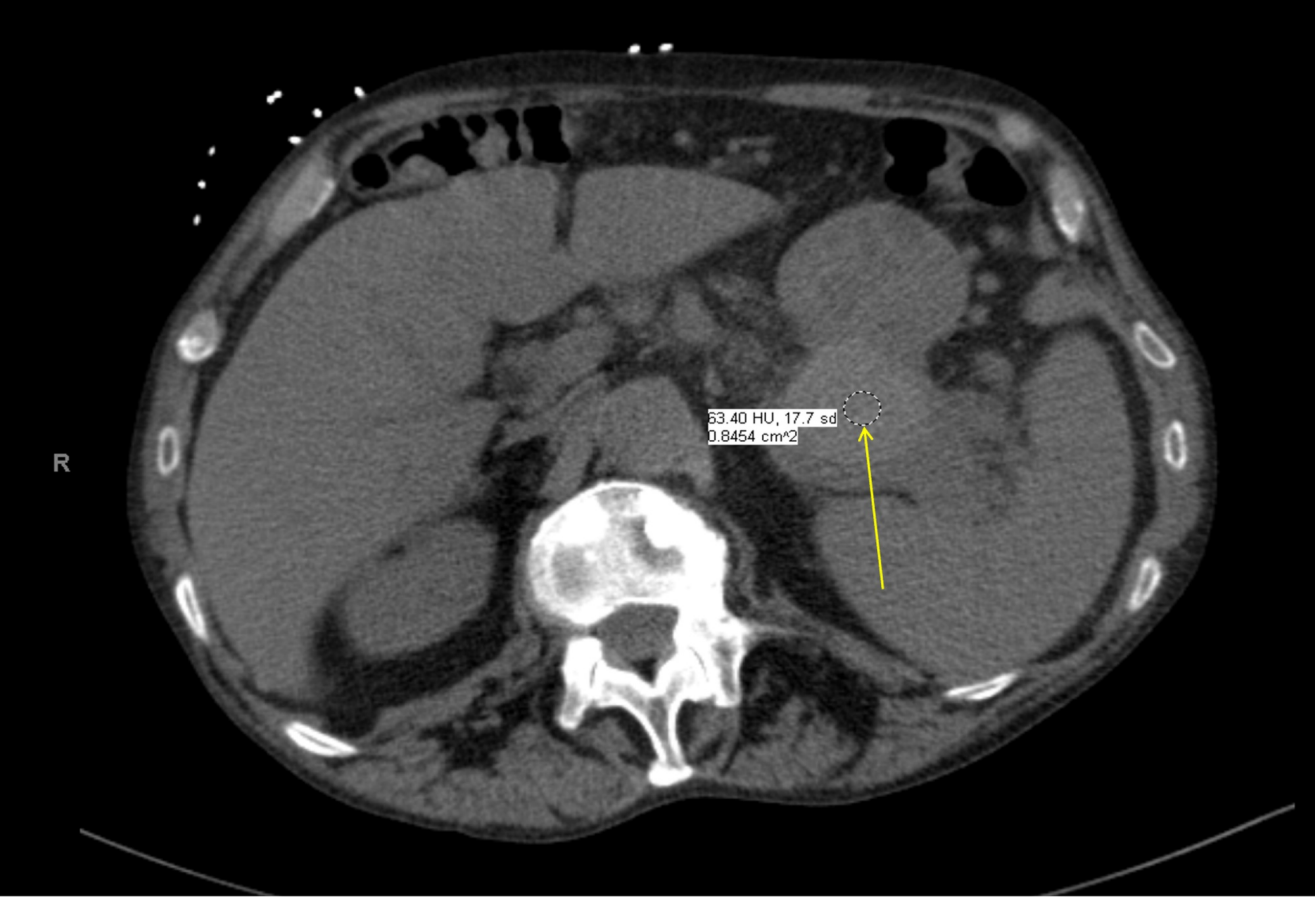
CT scan of the abdomen with peri-pancreatic stranding and multiple small fluid collections The arrow points to the largest collection in the superior aspect of the pancreatic tail measuring 4.7 x 3.4 cm.
